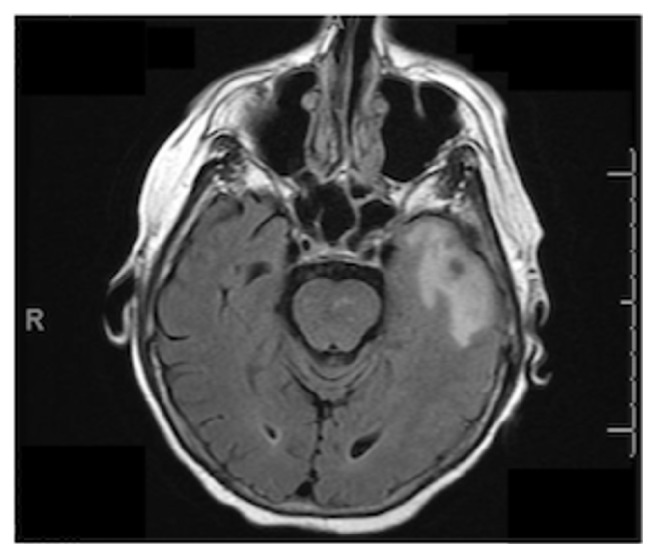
Axial flair MRI showing vasogenic edema of the temporal lobe.
